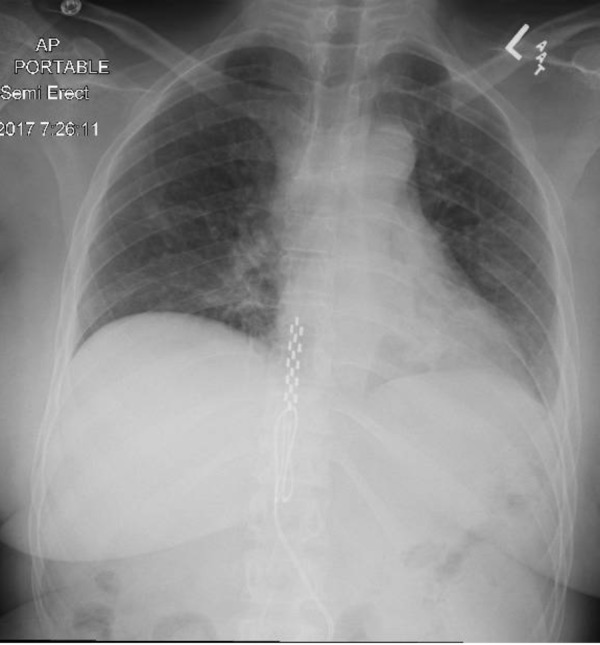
Chest X-ray before the surgery showing no pleural effusion.
